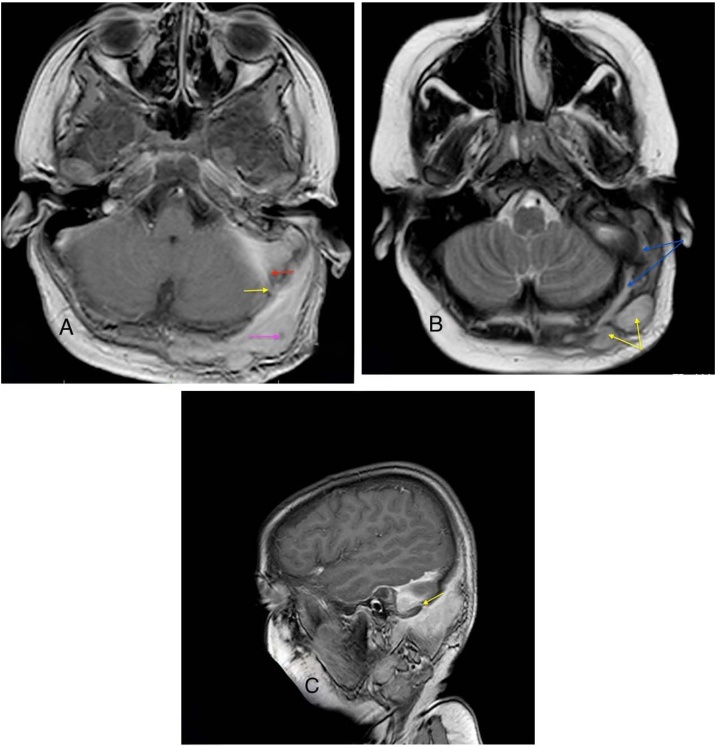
(A) T1WI MRI post contrast axial showing sigmoid sinus thrombosis (red arrow) and scalp abscess (pink arrow) connected with emissary vein (yellow arrow). (B) T2 WI axial MRI showing long sinus tract extended from the mastoid cavity to the soft tissue of left occipital region (blue arrow) connecting multiple abscess (yellow arrow). (C) Post contrast Sagittal T1WI MRI showing communication between infected sigmoid sinus and scalp lesion through emissary vein (yellow arrow) across the mastoid foramen.
